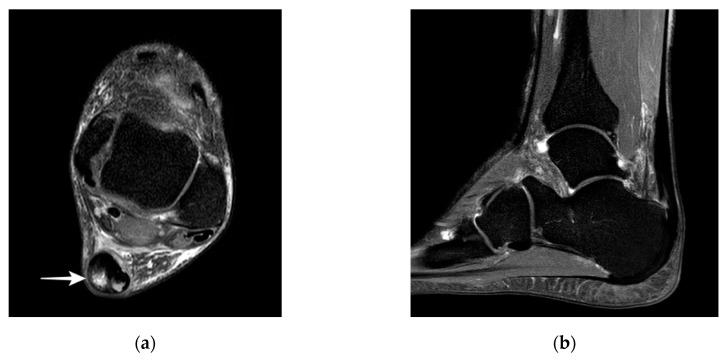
MR images of partial Achilles tendon ruptures: (a) partial rupture in the dorsal part of the midportion; (b) partial rupture in the insertion. MR; magnetic resonance.
